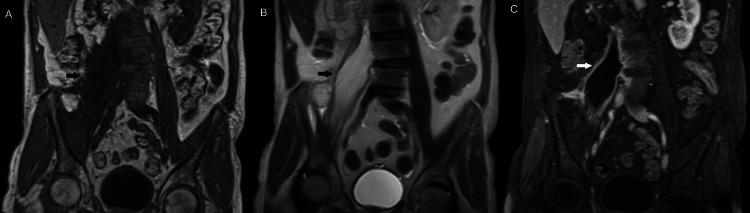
A 69-year-old diabetic man with pyelonephritis. (A-C) Coronal T1- (A), T2- (B), and enhanced, fat-saturated T1-weighted (C) MR images display intramuscular abscess (arrow) of the psoas muscle.
